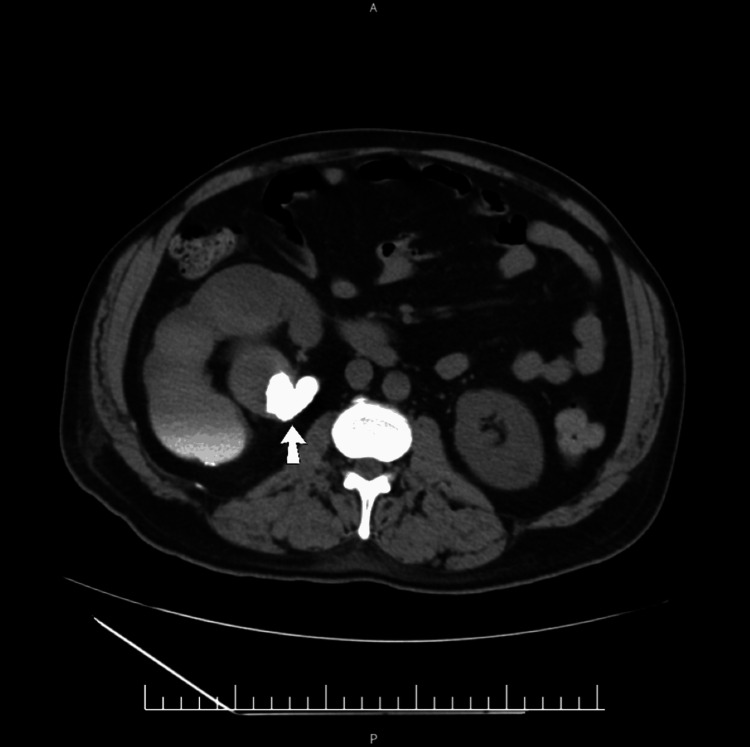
Computed tomography of the kidneys, ureters, and bladder (KUB). Contrast-enhanced computed tomography (KUB, axial view) demonstrating an enlarged right kidney with a large, highly attenuated calculus (arrow) obstructing the right pelviureteric junction. This has resulted in severe parenchymal thinning and hydronephrosis.
